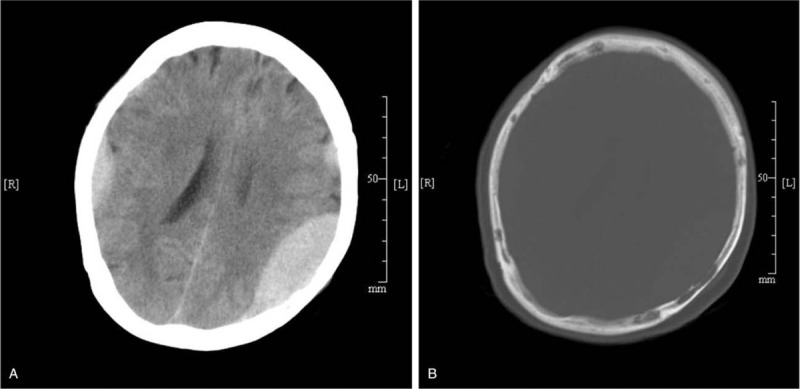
(A) Computed tomography revealed a 6 × 3 × 6.5-cm, epidural, slightly dense mass in the left parietal region and 2 smaller epidural masses in other regions. The left mass was compressing part of the brain, the left ventricle was smaller than the right ventricle, and the midline was not shifted. (B) The skull showed patchy osteolysis, and there were small lytic changes.
